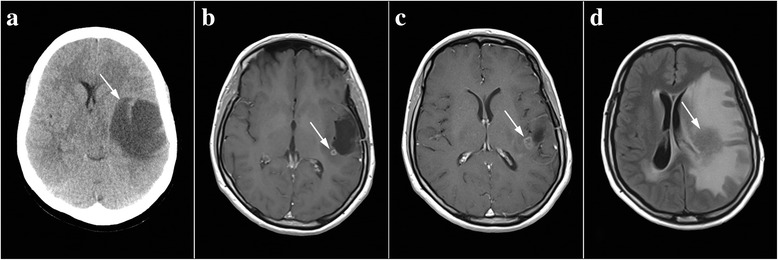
Overview of control scans up to the introduction of PT. Tumor tissue is indicated by arrows. a CT scan of a diffuse astrocytoma. b The NMRI after the completion of oncological treatment indicated a recurrence measuring 5 mm. c Recurrence 11 mm. d Progression of the tumor
